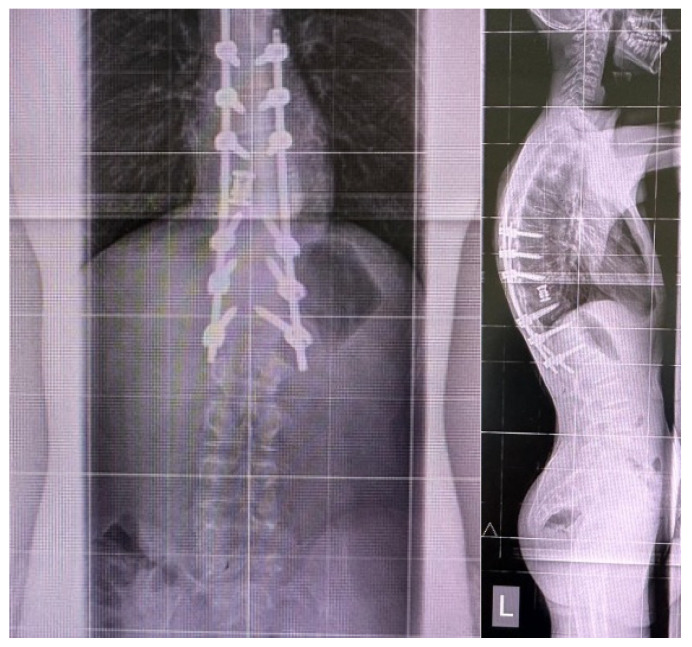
Postoperative radiograph scans demonstrating successful correction of sagittal and coronal deformity with restoration of thoracic alignment and spinal balance. Anterior vertebral body reconstruction and posterior instrumentation remained in satisfactory position with adequate spinal canal decompression.
